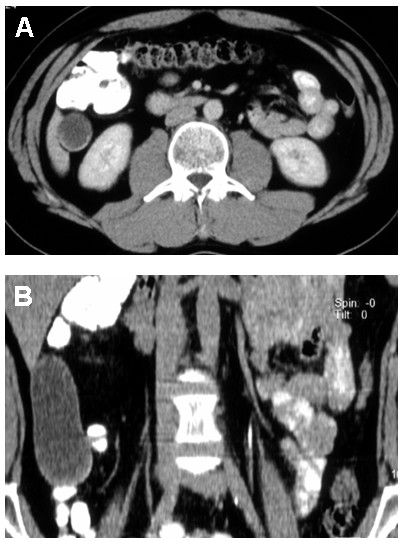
Contrast-enhanced computed tomography of the abdomen reveals a well-delineated mucinous mass with curvilinear calcification. A) The axial view indicates that the mass is located at the inferior tip of the right liver lobe. The surrounding fat tissue does not show any fat-stranding. B) The coronal view clearly shows the cranio-caudal extension of the mass from the liver segment six to the ileocaecal area.
